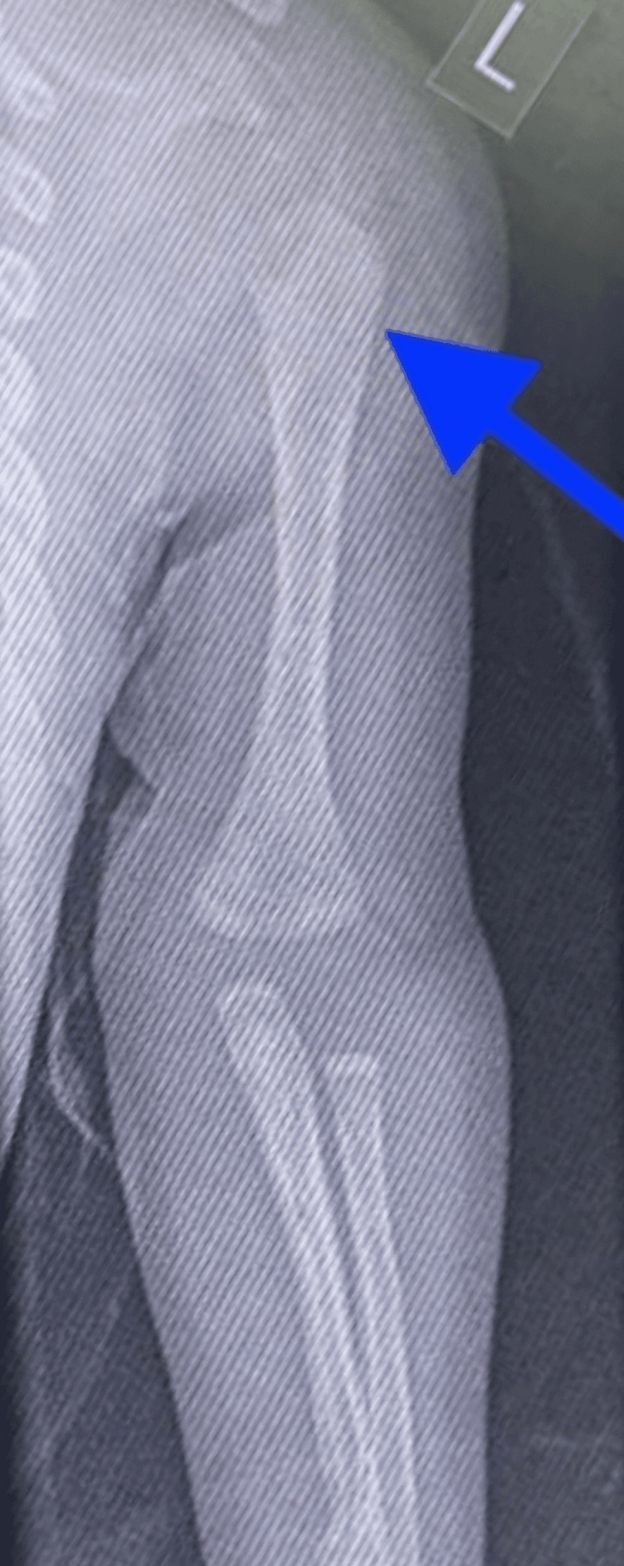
X-ray scan shows area of destruction in the proximal shaft of the left humerus.
